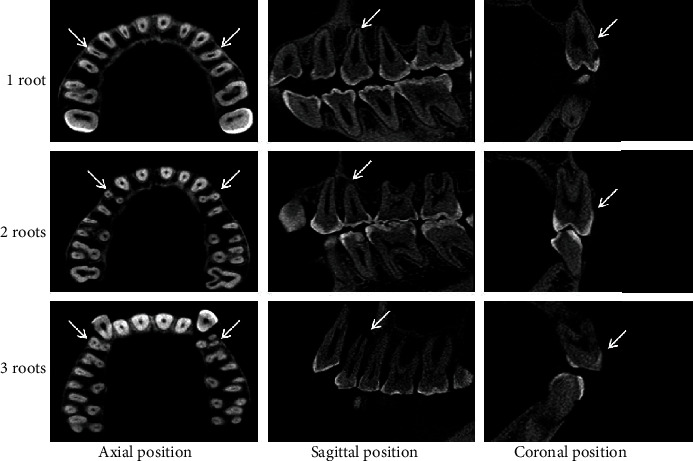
CBCT images showing root types of maxillary first premolars. Arrows indicate the examined teeth.
